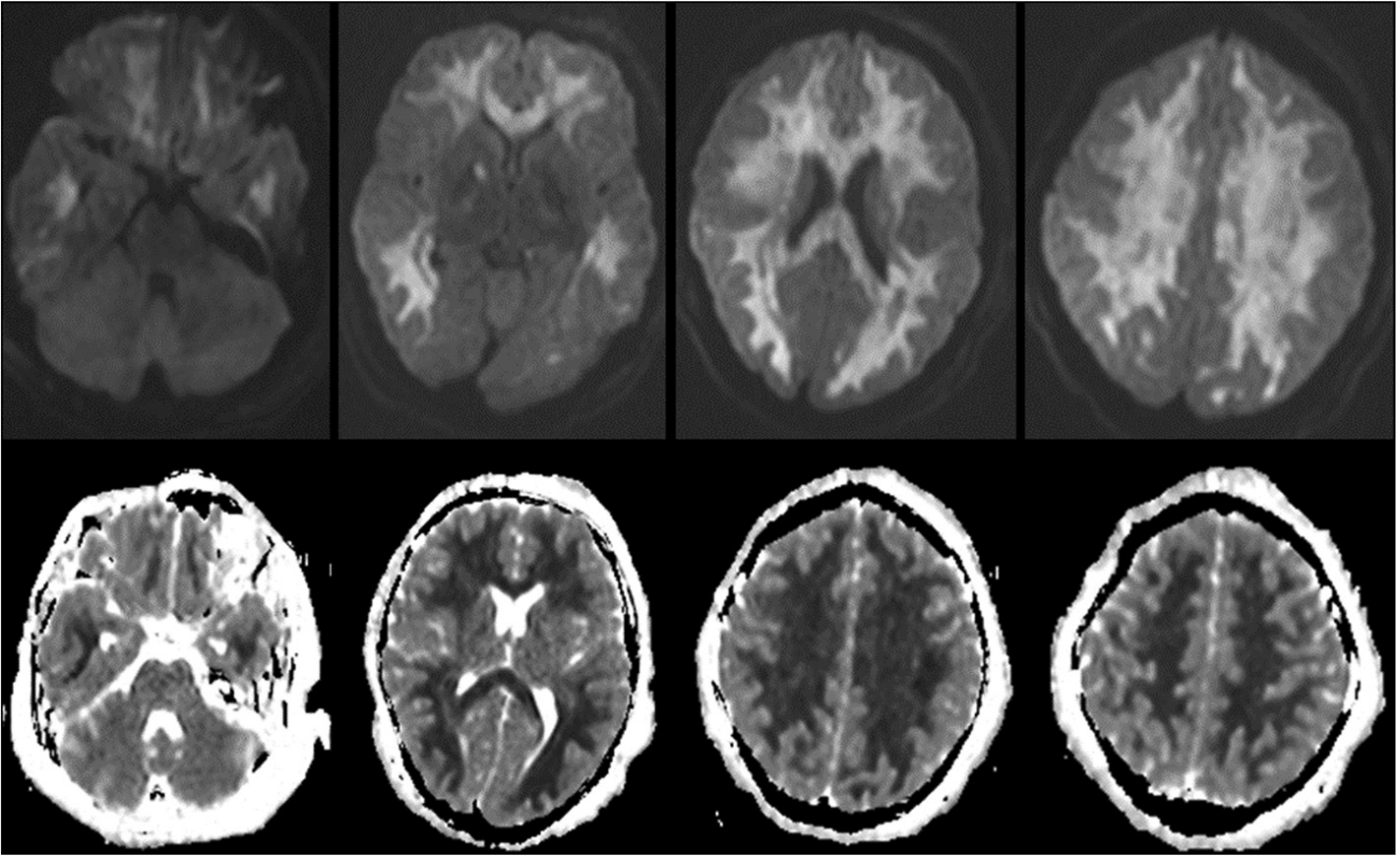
Diffusion weighted images (DWI). DWI in the upper row and apparent diffusion coefficient (ADC) maps in the lower row show areas of diffuse and symmetric diffusion restriction in the cerebral subcortical white matter and right globus pallidus that is corresponding to the changes seen in FLAIR images. Note the sparing of infratentorial structures.
